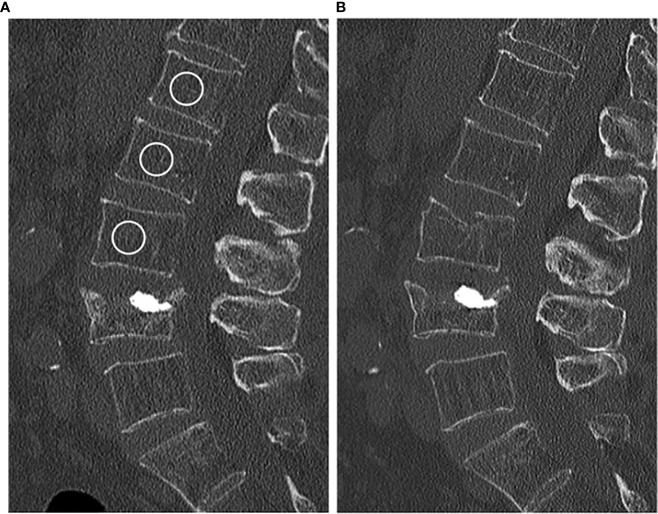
(A) Baseline CT scan of a 84-year-old female. The circles in vertebrae L1–3 represent the ROIs used for calculating the BMD (in this case 75.1 mg/cm^3^). (B) New vertebral compression fracture of L3 in the same patient 11 months later.
